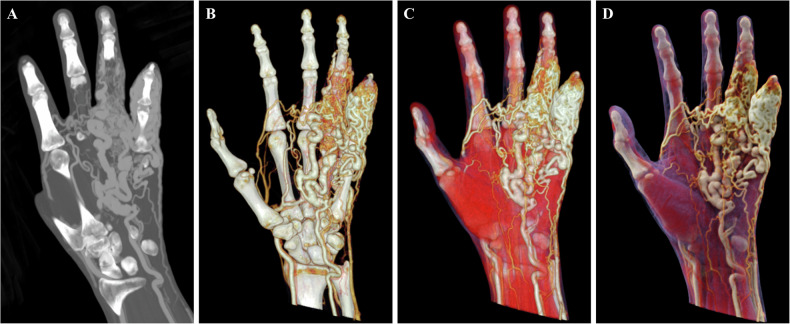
Hand arteriovenous malformation in a 39-year-old male. A The CTA-based MIP image reveals a tortuous vascular nidus involving the 3rd to 5th digits of the left hand; however, severe spatial overlap hinders accurate assessment. B VR reconstruction of the same patient demonstrates multiple tortuous pathological vessels. C, D CR images of the same patient, using varying threshold ranges, clearly delineate the spatial relationships among the vascular lesion, surrounding soft tissue, and bone, providing photorealistic 3D anatomical visualizations
